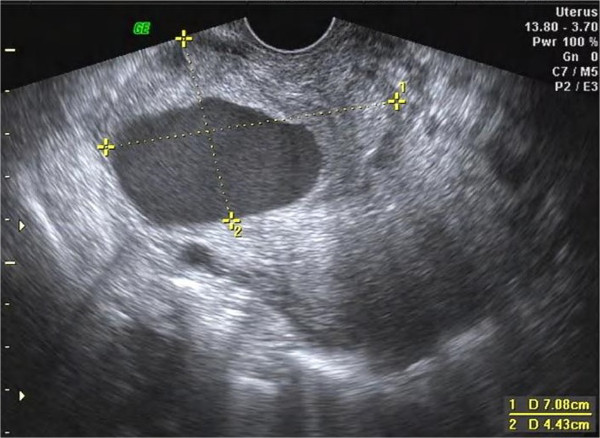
Two-dimentional ultrasound image of the pseudoaneurysm. The pseudoaneurysm is visible in the anterior wall of the lower part of the uterus. The wall varied in thickness and the luminal surface of the wall is uneven.
